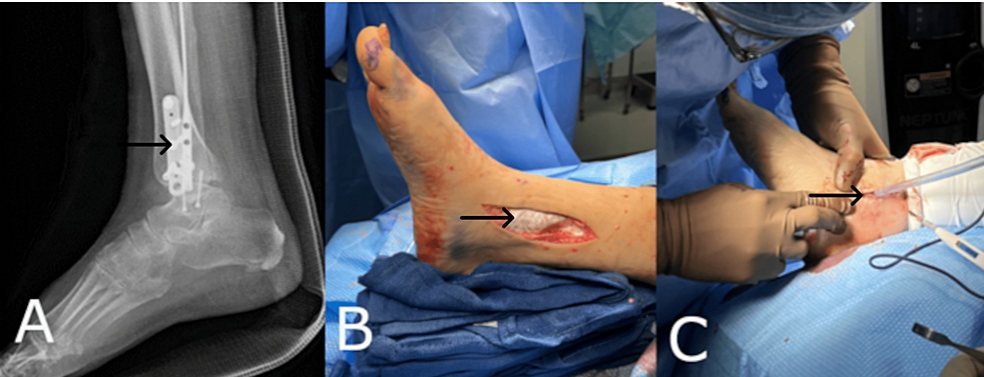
(A) Radiographic image of the ankle post open reduction and internal fixation procedure. (B) The synthetic hybrid-scale fiber matrix applied to the surgical wound bed and sutured in place. (C) The incision and drainage procedure performed after the development of the surgical site infection. The synthetic hybrid-scale fiber matrix prevented the exposure of deep hardware.
